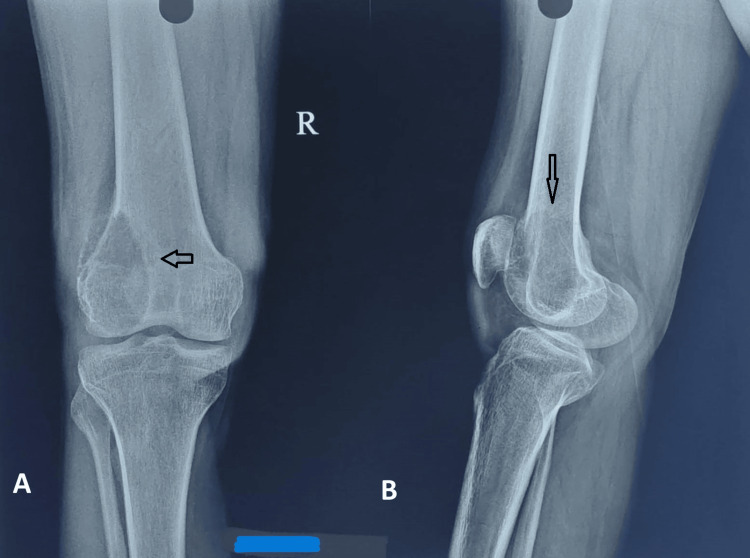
(A) Anteroposterior plain radiograph of the right knee joint showing a lytic lesion involving the right lateral condyle of the distal femur, with the arrow showing the margins of the lesion. (B) Lateral plain radiograph of the right knee joint, with the arrows showing the lesion in the right distal femur in both the viewss.
